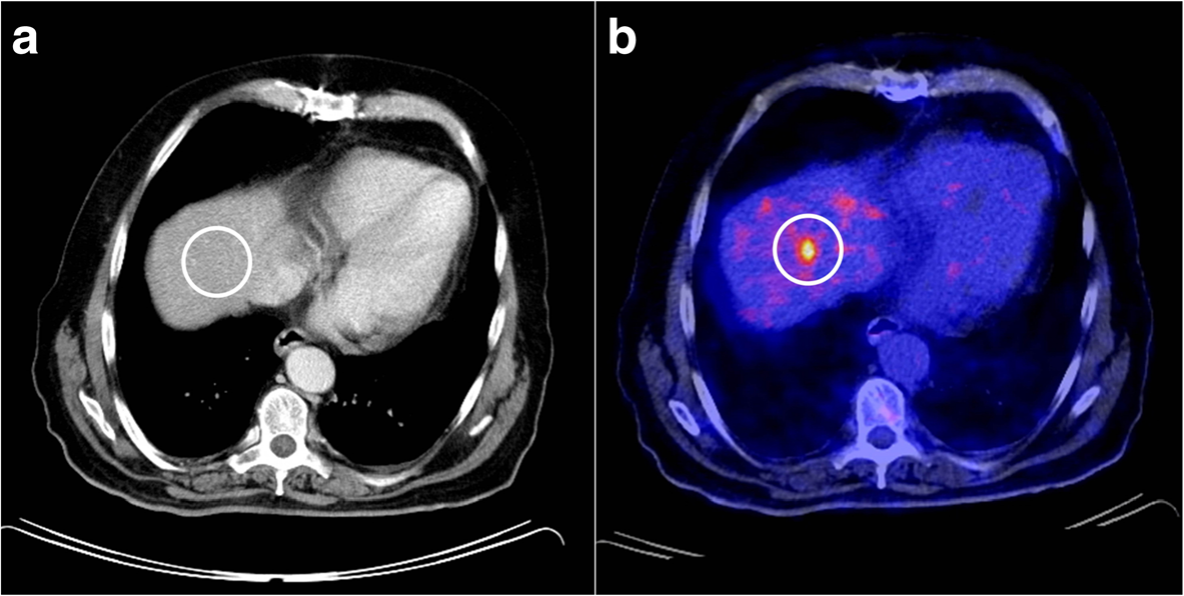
CT and PET/CT for hepatic imaging in anorectal malignant melanoma. A sixty-three-year-old male patient (*patient 2*) with a large anorectal melanoma, inguinal lymphatic metastases on both sides, and distant metastases. PET/CT depicts two hepatic metastases in segment six (b) and eight (*not in the picture*) without any corresponding findings on contrast-enhanced CT (a). Staging based on CT underestimated tumor stage by missing the distant metastatic spread completely in this patient
